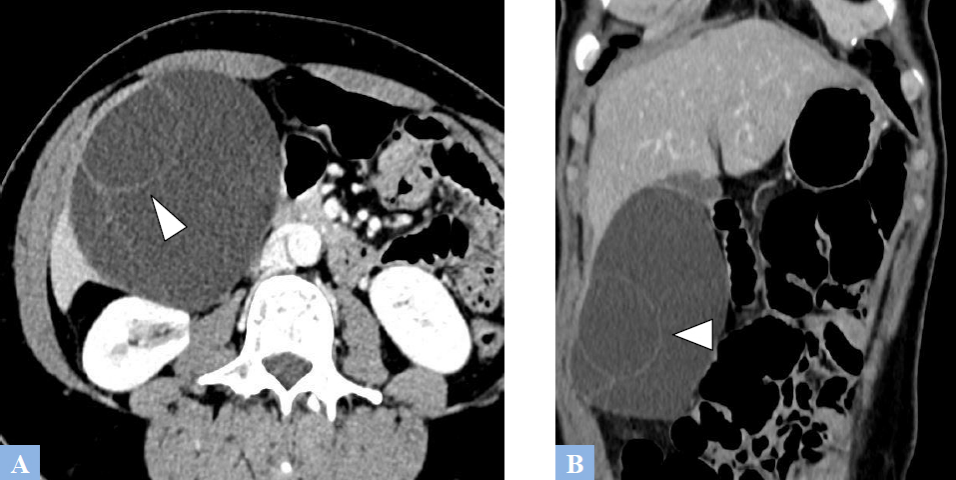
Low-grade mucinous cystic neoplasm (formerly mucinous cystadenoma) in a 37-year-old female patient presenting with abdominal heaviness. A. CT image in the axial plane at portal phase of enhancement. Rounded, exophytic cystic lesion in segment V, well-defined, containing thin septations enhanced after contrast injection (arrowhead). B. Same study, coronal reconstruction showing intracystic septations enhanced after contrast injection (arrowhead).
